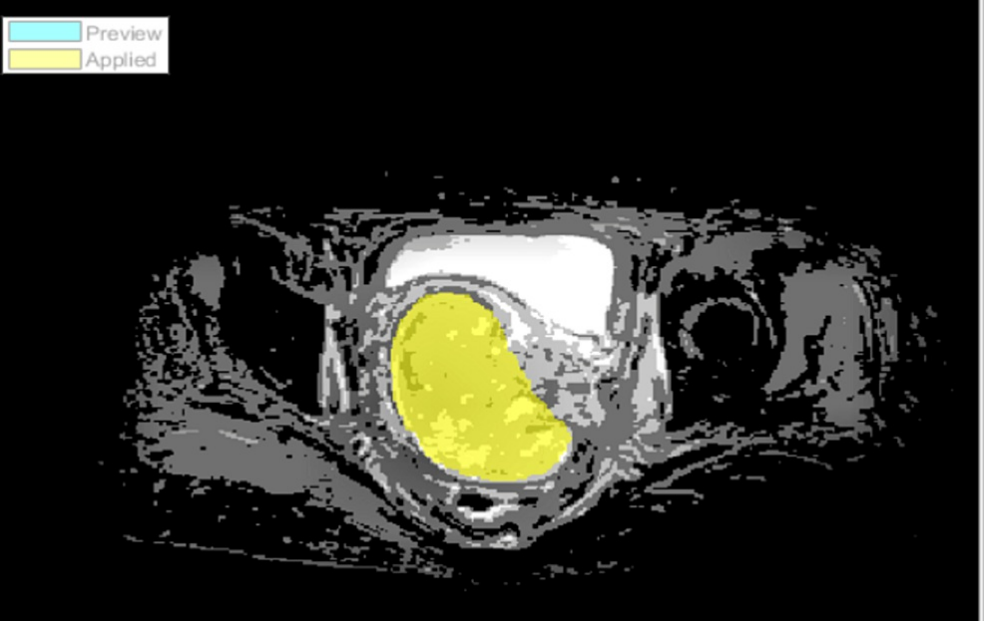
Semi-automated segmentation with CLAHE enhanced on axial plane of cervical DWI-MRI image
